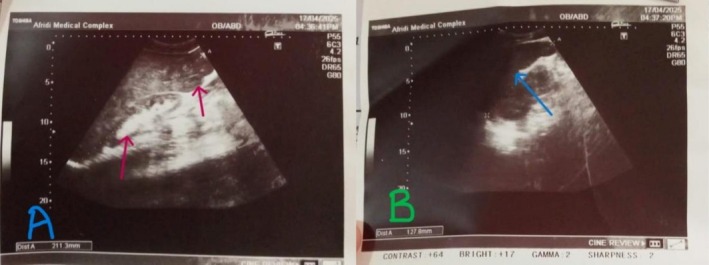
Ultrasonography of the abdomen, demonstrating hepatosplenomegaly. Ultrasound image revealing significant hepatomegaly (Figure 5A) (liver span 21.1 cm) with homogenous parenchymal echotexture. The spleen (Figure 5B) measures at the upper limit of normal (12.7 cm).
